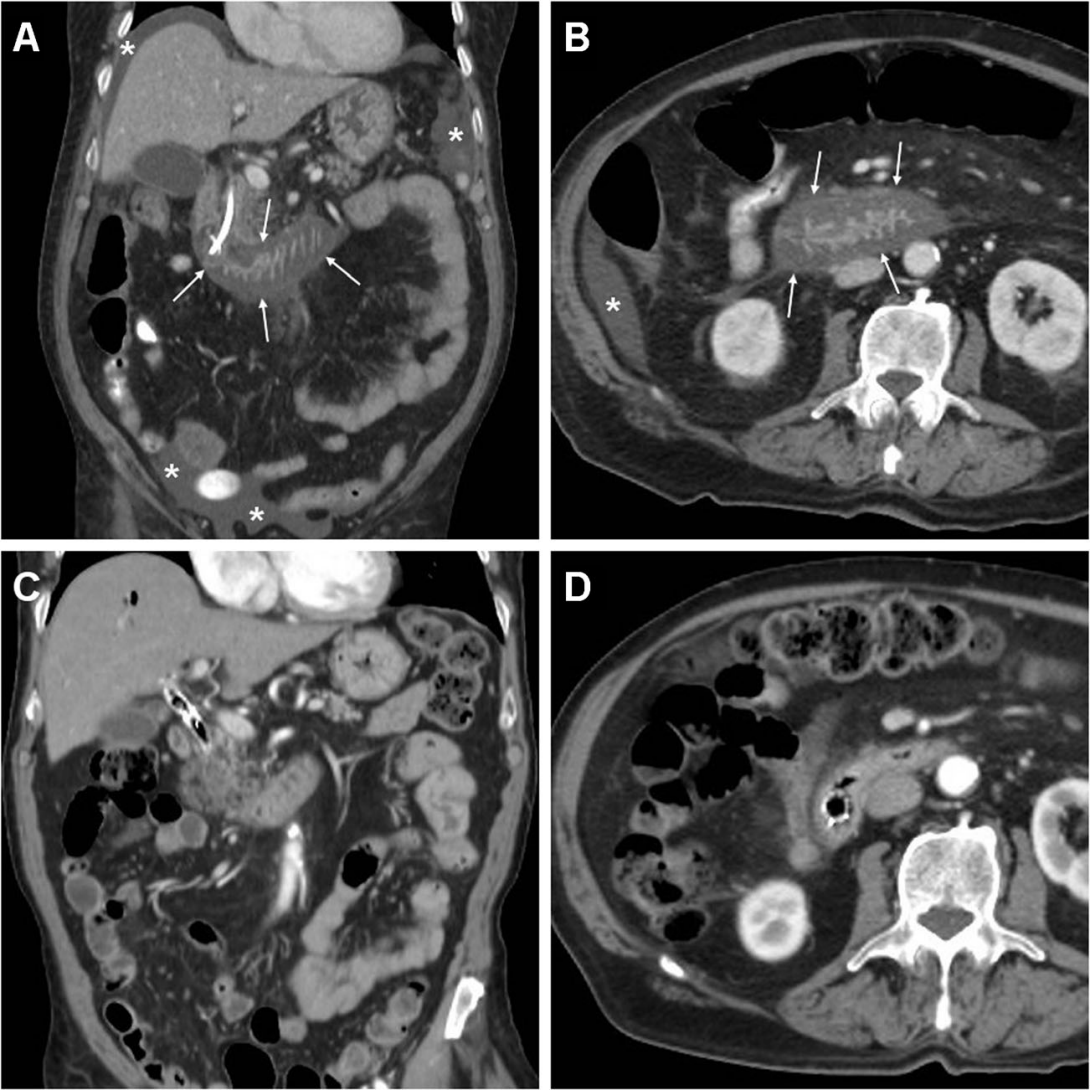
Acute reversible duodenitis following ERCP. A 78-year-old male with common bile duct (*CBD*) carcinoma causing concentric stenosis of the proximal choledochus suffered from acute diffuse abdominal pain without peritonitis 24 h after ERCP including cytological brushing and positioning of a 10-French plastic biliary stent. Urgent contrast-enhanced CT (a, b) showed a correctly placed biliary stent, appearance of moderate ascites (*) and of marked circumferential thickening of the duodenum from the Vaterian papilla to the Treitz angle, with enhancing mucosa and hypoattenuating oedematous submucosa (“*target sign*”, *thin arrows*), and minimal associated inflammatory changes in the periduodenal fat. Imaging findings, laboratory data and the subsequent course excluded iatrogenic pancreatitis, haemorrhage and DP. Three weeks later, the plastic biliary stent was removed and replaced with a self-expanding metal stent (*SEMS*). Follow-up CT (d) showed imaging resolution of both biliary obstruction and acute duodenal inflammatory changes [partly reprinted from Ref. 20]
